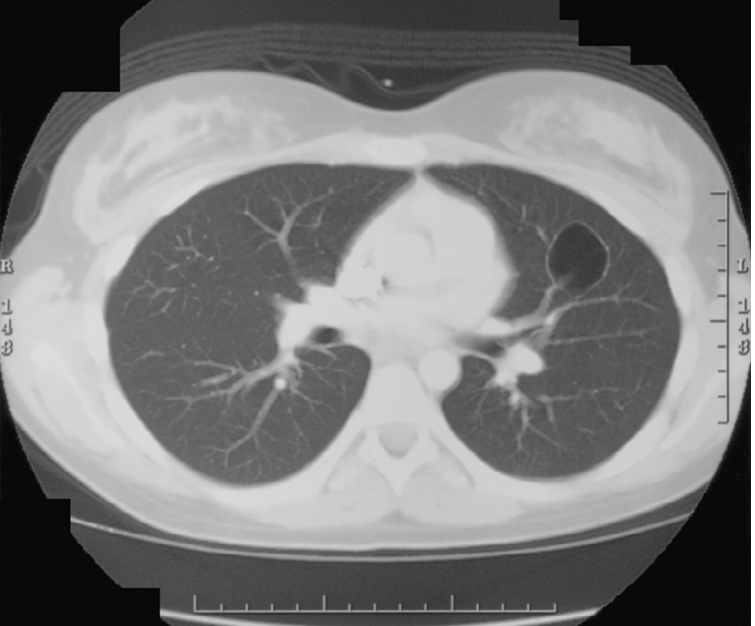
Follow-up (12 mo.) chest CT scan in the same patient on maintenance 6-mercaptopurine therapy with a residual cavitary lesion within the lingual of the left lung.
